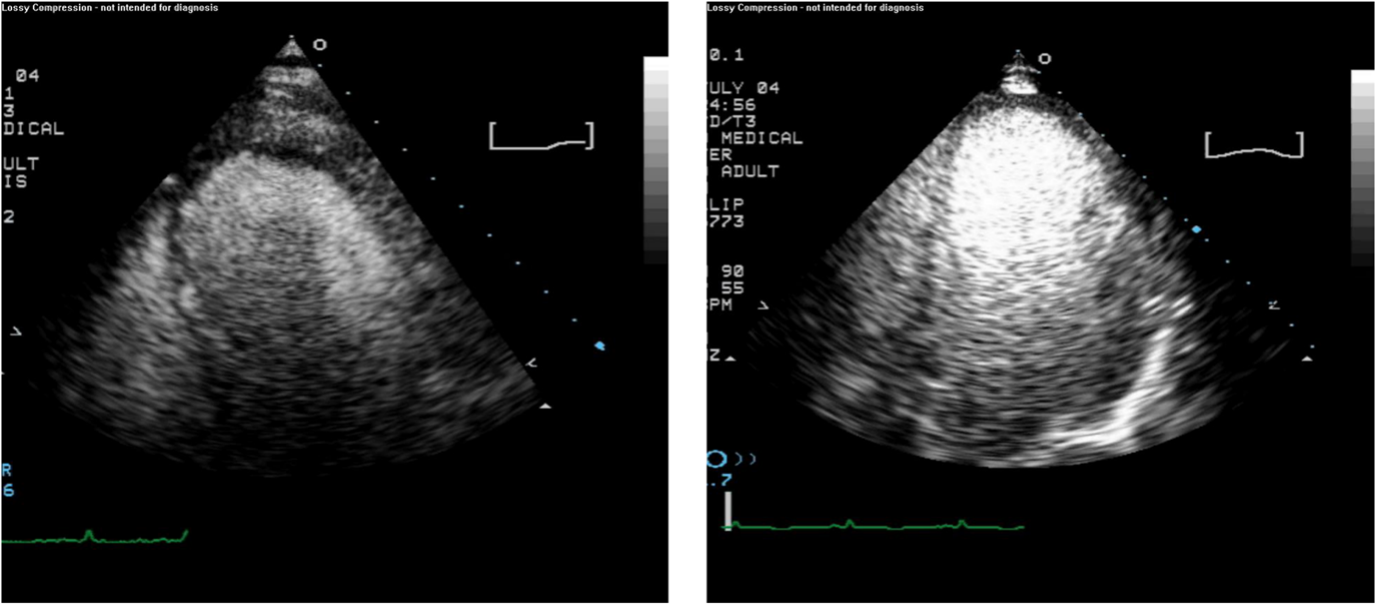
Left panel demonstrates myocardial contrast echocardiogram in a patient with an anterior myocardial infarction in whom virtually no apical opacification is seen indicative of nonviable myocardium. The panel on the right shows another patient with an anterior apical myocardial infarction in whom at least partial apical opacification exists suggesting myocardial viability.
